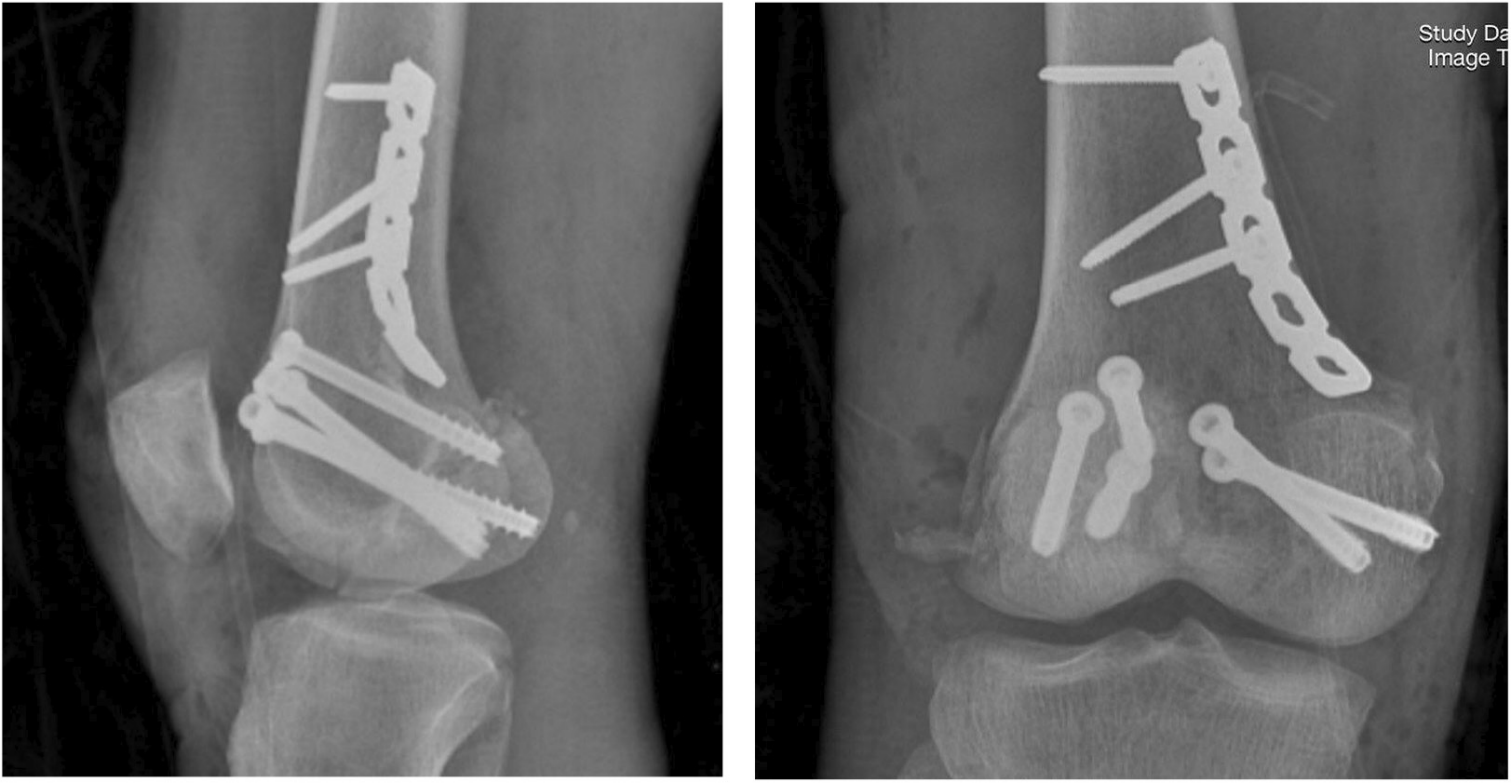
Postoperative radiograph of the right knee in anteroposterior and medio‐lateral views with cannulated cancellous screws.
